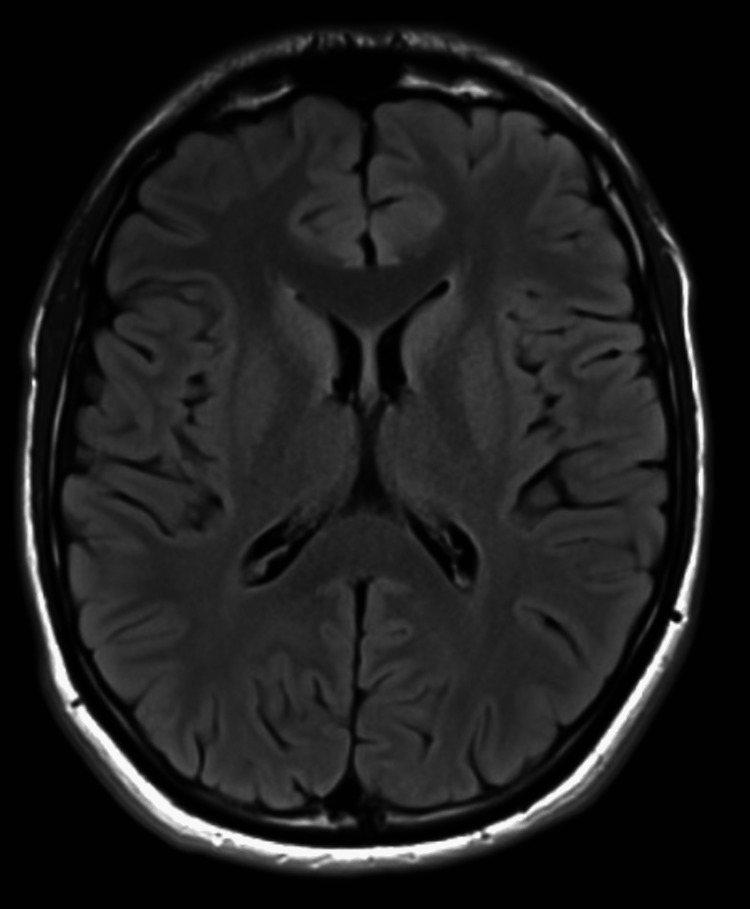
Brain MRI with no abnormal signal intensity
